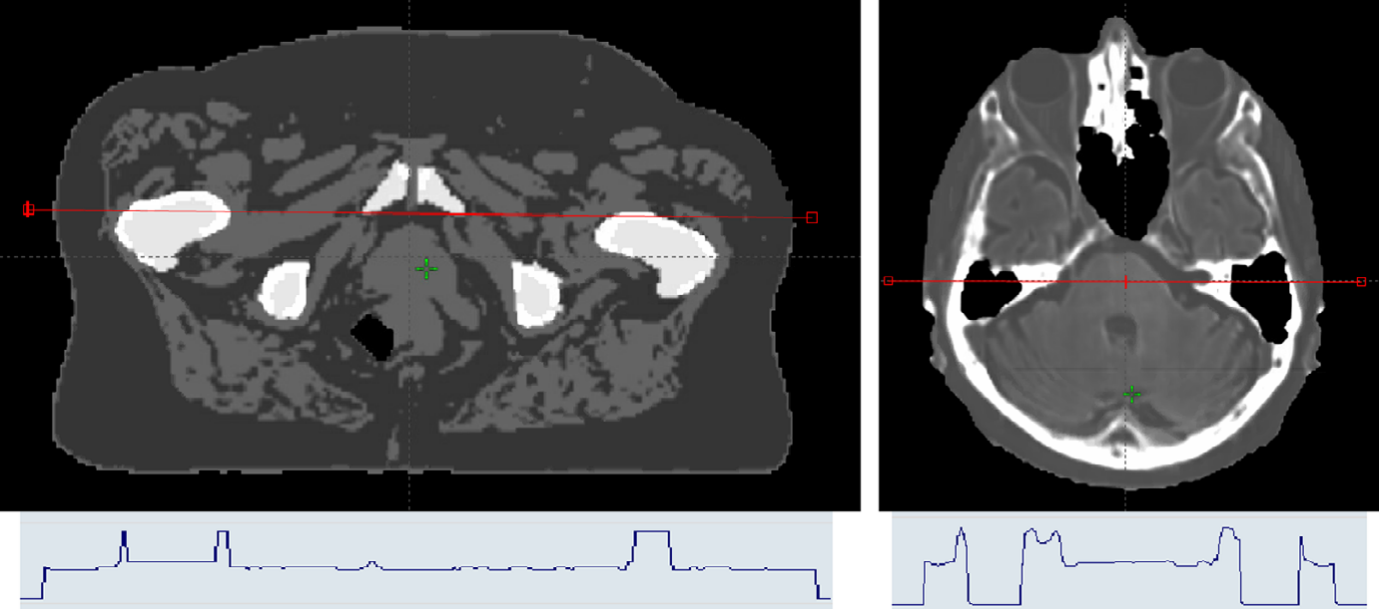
Example of s‐CT images obtained for the pelvis (Left) and the brain (Right) with a HU profile (red line on the s‐CT) stressing the segmented (left) and continuous (right) HU affectation.
